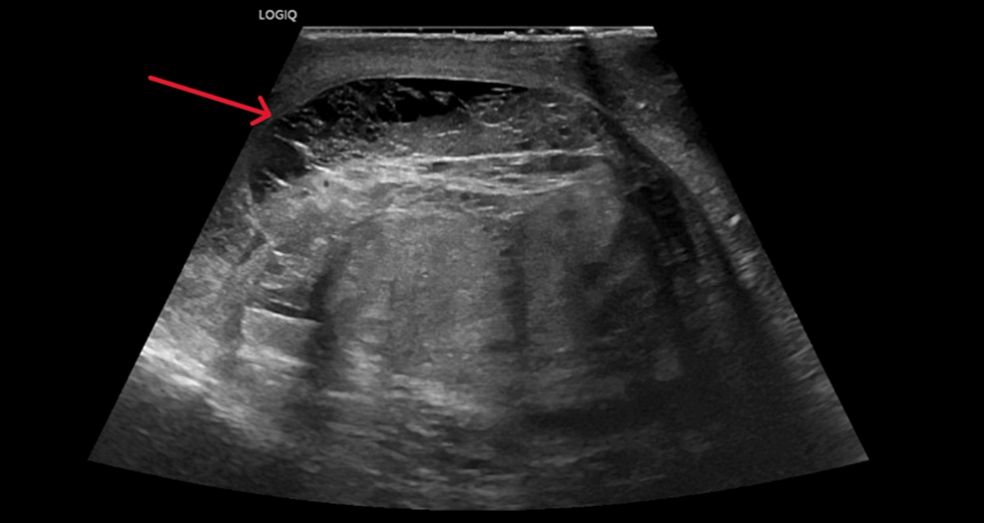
Follow-up sagittal grayscale ultrasound of the right testicle three days after injury. Hematoma is stable from prior examination (red arrow) and heterogenous echotexture are still present on this examination.
